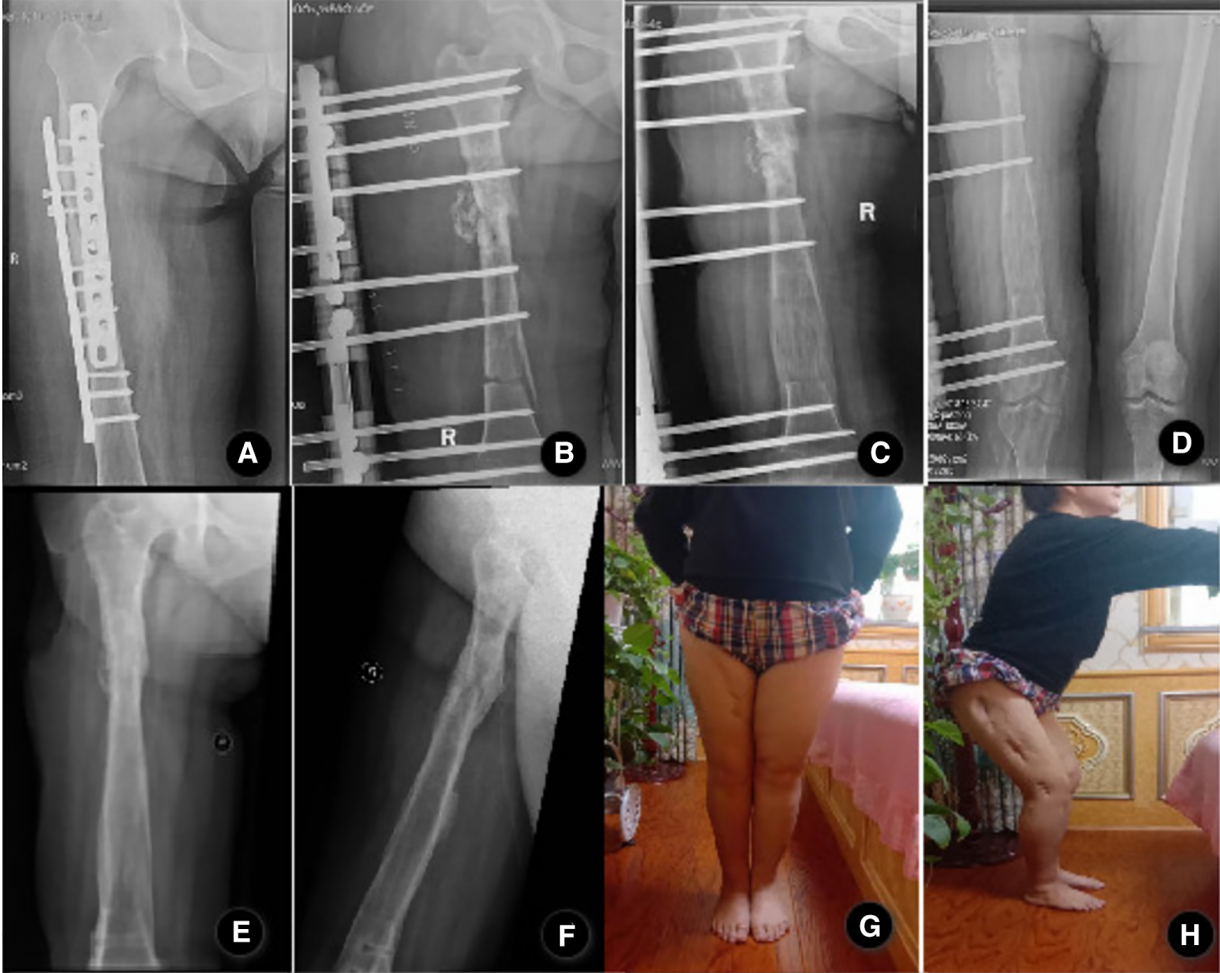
A 39-year-old female patient with a right femur fracture caused by a fall accident and post-traumatic osteomyelitis after plate internal fixation. (A) Anteroposterior x-ray showed osteomyelitis of the femur; (B) Anteroposterior x-ray films on the day after radical debridement and installation of a rail fixator system, the patient underwent the acute shortening and re-lengthening with the Ilizarov technique after resection of the infected zone, right lower limb was 7.9 cm shorter than the left after surgery; (C) Anteroposterior x-ray films at 98 days after the operation, bone healing was observed on both sides of acute shortening and compression. (D) Anteroposterior x-ray films at 176 days after the operation, both lower limbs equal in length without shortening. (E,F). Anteroposterior and lateral x-ray films showed good bony union and good regenerate consolidation at 12 months after the removal of external fixation;(G.H)The patient showed a good function of flexion and extension of the knee joint;.
